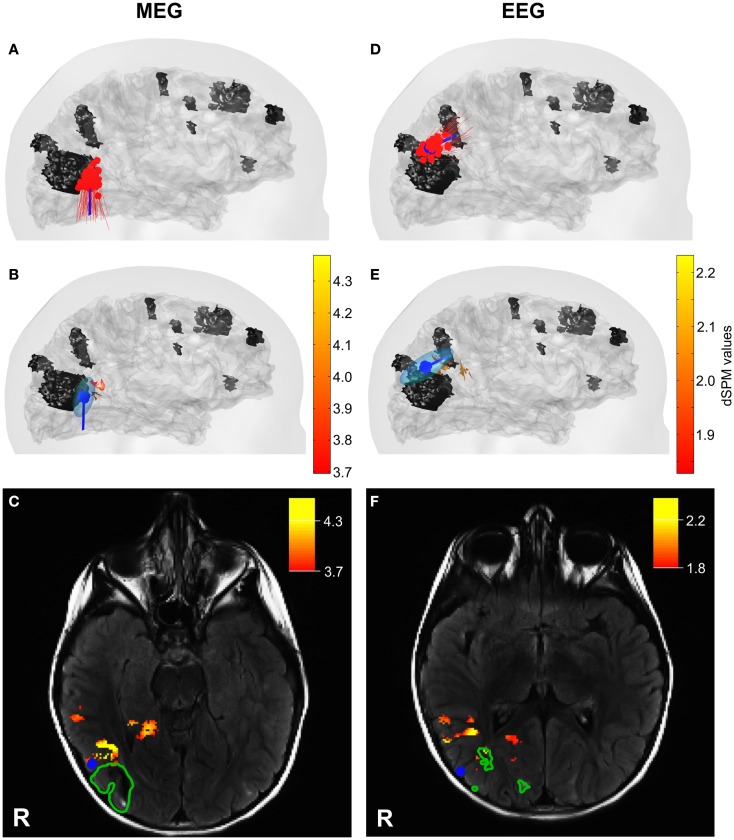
ECD and MNE localizations for interictal MEG (left column) and EEG (right column) spikes. (A,D) Lateral views on the head model with ECD localization for each interictal spike (red) and the averaged interictal spikes (blue). (B,E) Lateral views on the head model with MNE localizations (red – yellow scale) and ECD for averaged interictal spikes (blue) with ellipsoid representing the dipole-clusters. The tuber volumes are indicated in black. Skin and cortical surface are indicated in gray. (C,F) Axial slices of MRI FLAIR images with tuber margins (green), ECD localizations for averaged interictal spike (blue dot), and MNE localizations (red – yellow scale). R: Right.
